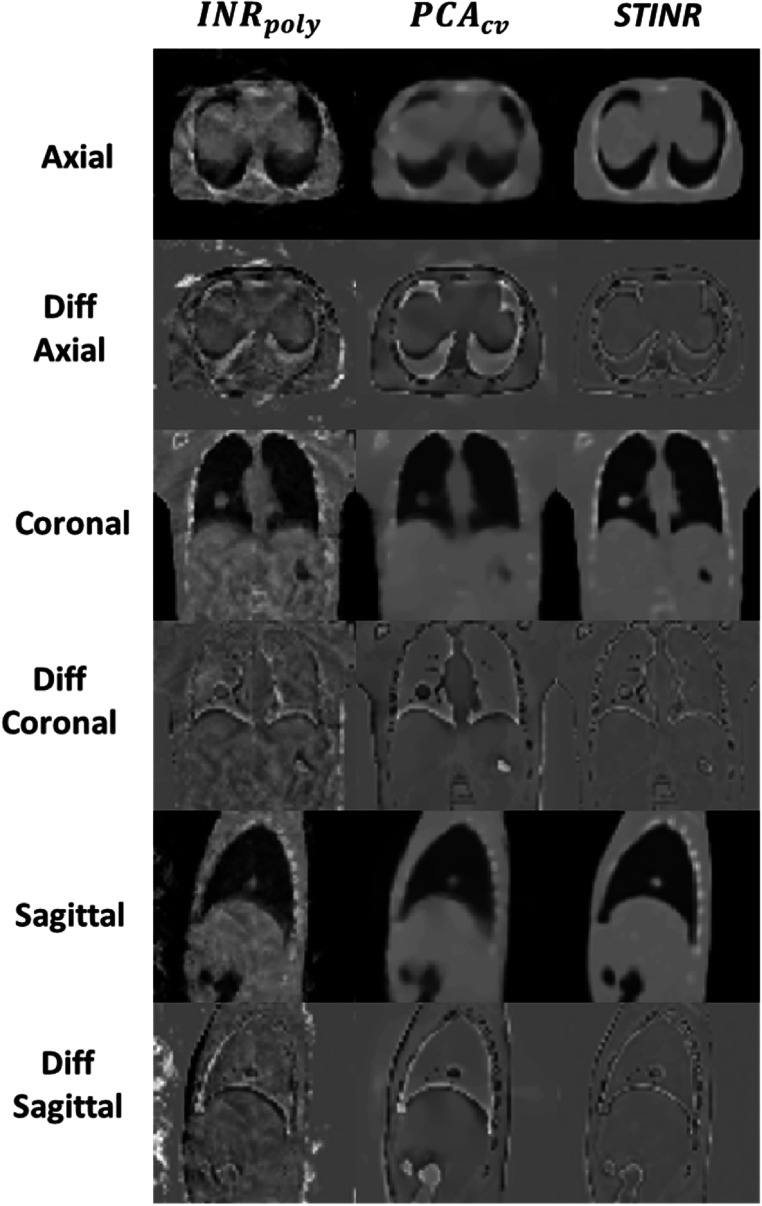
Comparison of the reconstructed CBCT volumes between $IN{R}_{poly},$
